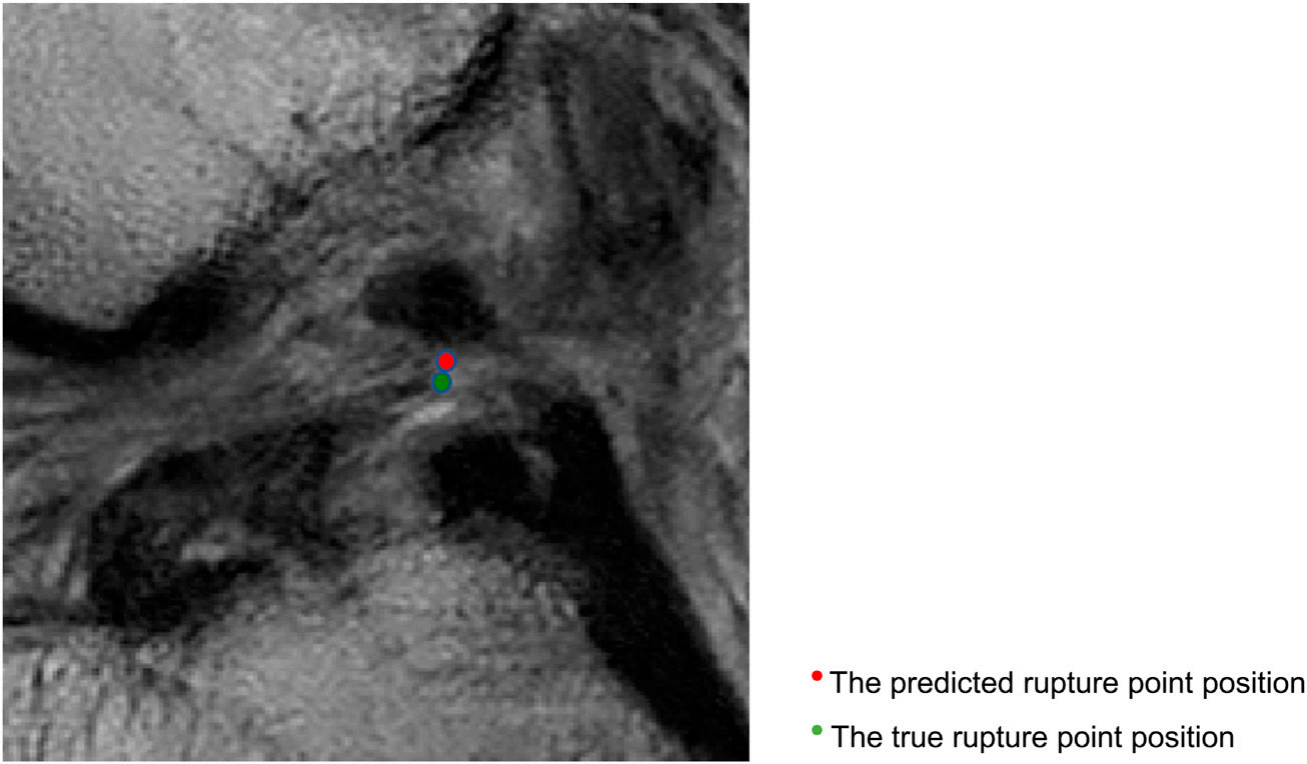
Sagittal views of the cropped MR image, correct localization and classification. The predicted rupture point is marked by red circle, while the true rupture point is green. The model predicted a correct localization, and the system shows a correct classification (the middle side).
